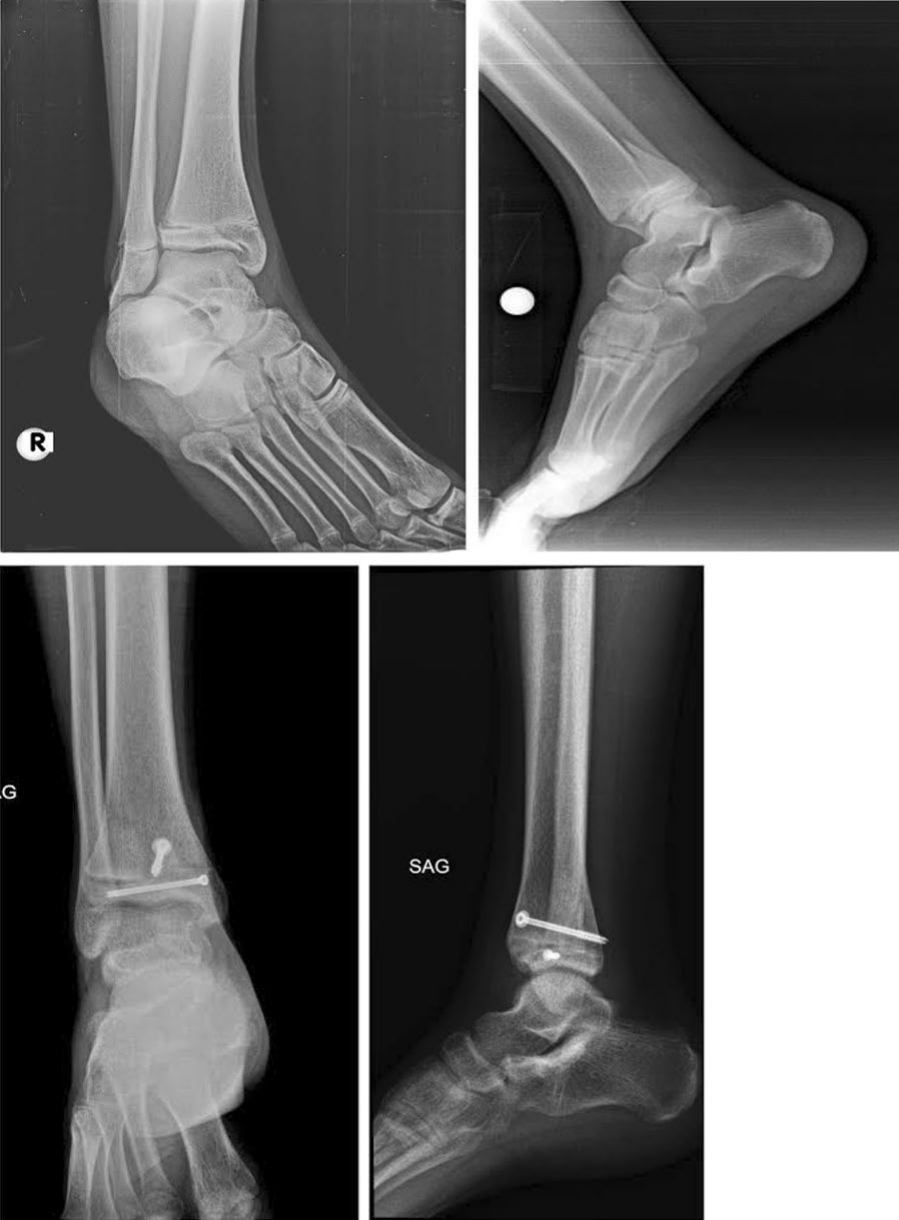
A 14-year-old boy with Triplane fracture. Pre-operative and post-operative X-rays after 3 months
